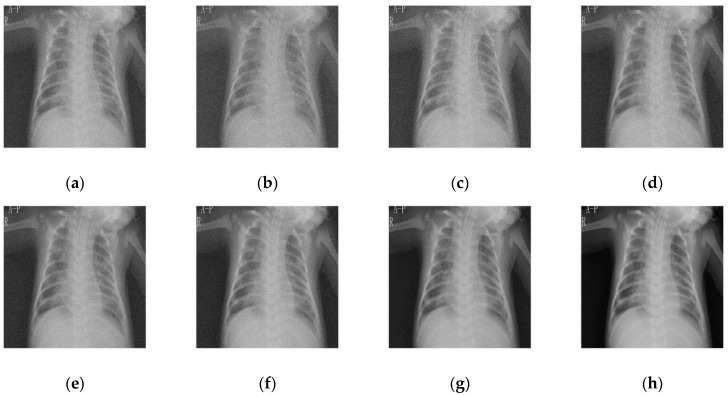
Denoised COVID-19 CT image 3 from the (a) [10], (b) [11], (c) [12], (d) [13], (e) [14], (f) [11], (g) [16], and (h) proposed frameworks, respectively.
